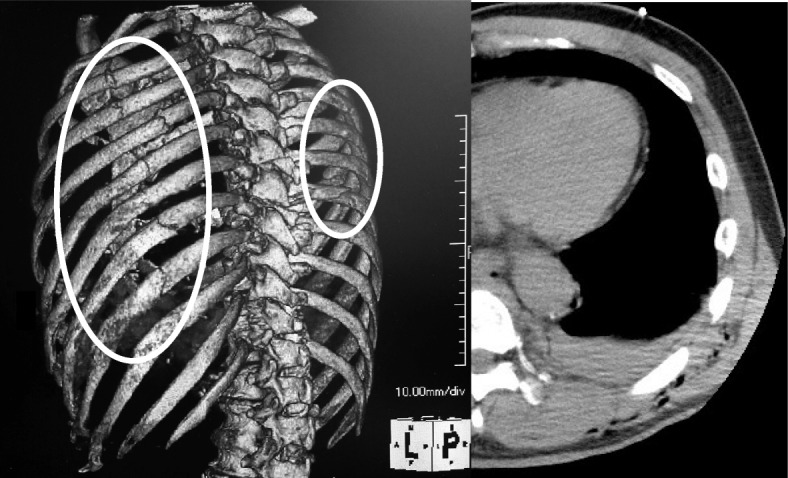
Plain computed tomography (CT) findings on admission. The left 3D-CT showed bilateral multiple rib fractures (circle), and there was no sign of pericardial injury on the right CT
